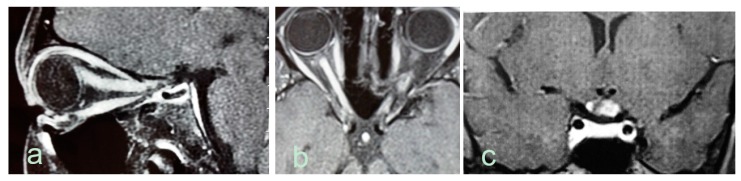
Optic nerve abnormalities on MRI in AQP4-seropositive NMOSD patients. (a). Sagittal T1-weighted MRI shows edematous gadolinium-enhancing optic nerve lesion extending from the eye to the intracranial segment. (b). Axial T1-weighted extensive gadolinium-enhancing lesion in both optic nerves. (c). Coronal T1-weighted MRI shows edematous gadolinium enhancing lesion in the optic chiasm.
